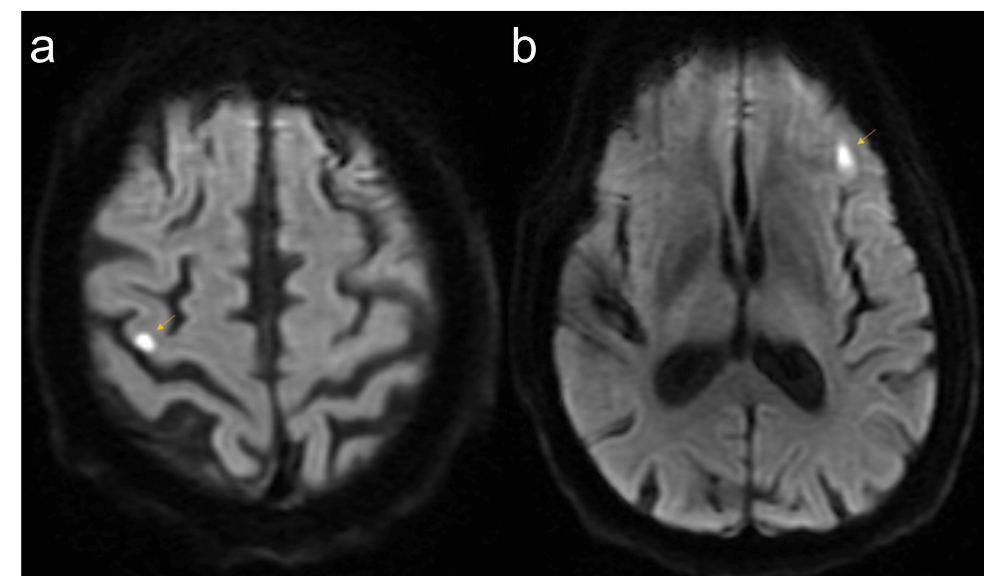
MRI brain without contrast, DWI, axial view. (a) Area of increased signal within the right frontal lobe in the region of the precentral gyrus (arrow) concerning for subacute infarction. (b) Area of increased signal diffusion within the left frontal lobe (arrow) concerning for subacute infarction. MRI: magnetic resonance imaging; DWI: diffusion-weighted imaging.
